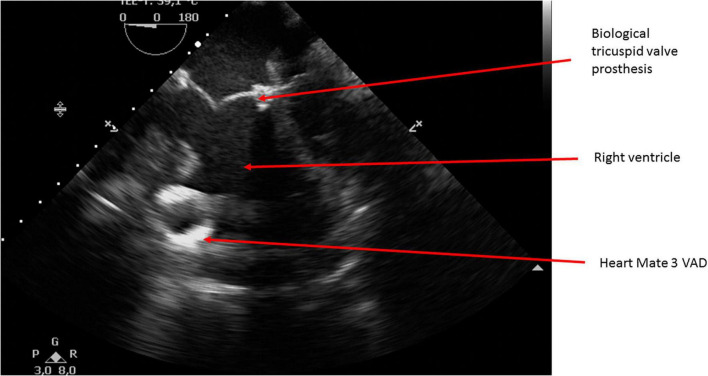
Transesophageal echocardiography showing pump position in the right ventricle at adequate distance from the tricuspid valve bioprosthesis.
